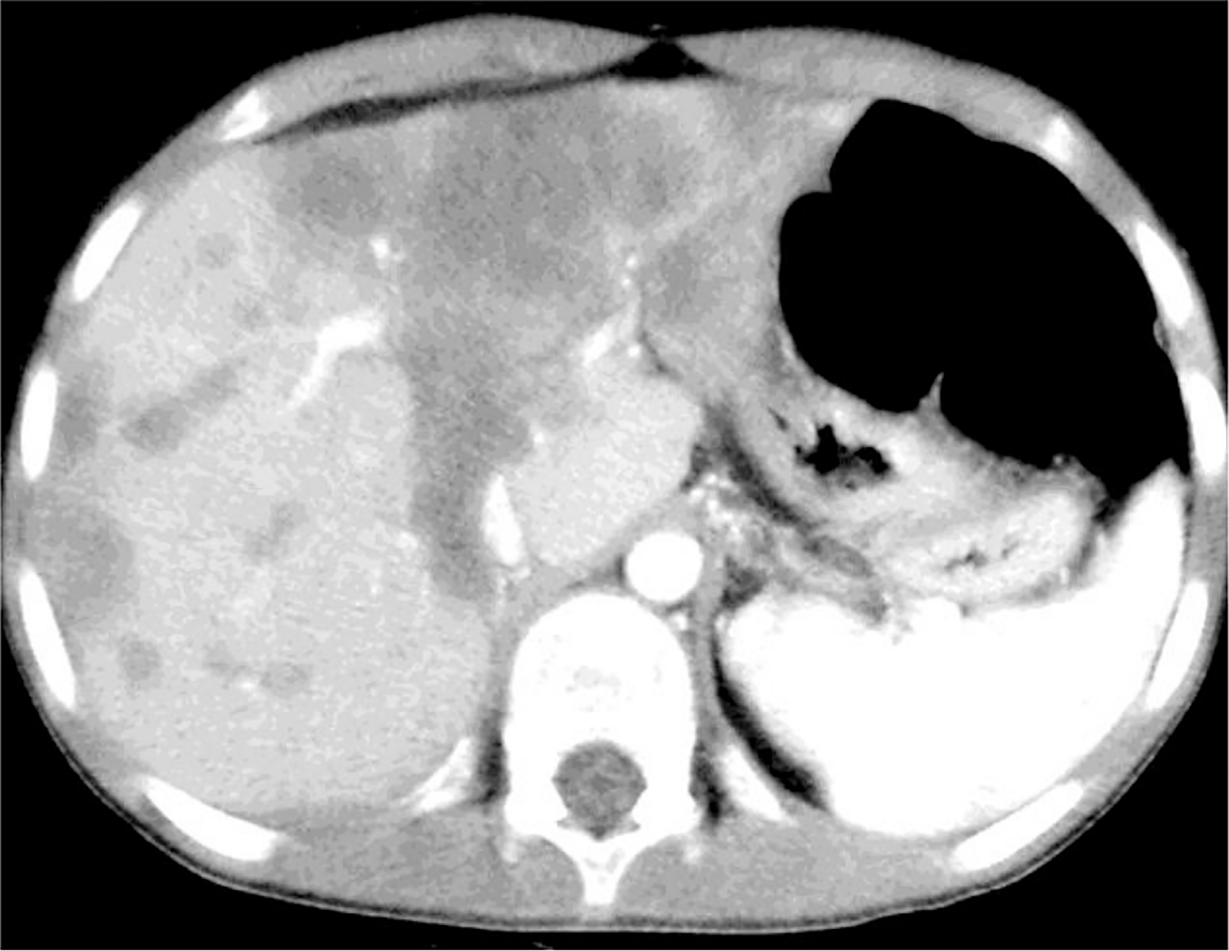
Radiological aspect of hepitalial hemangioendothelioma (HEHE) on CT scan.
